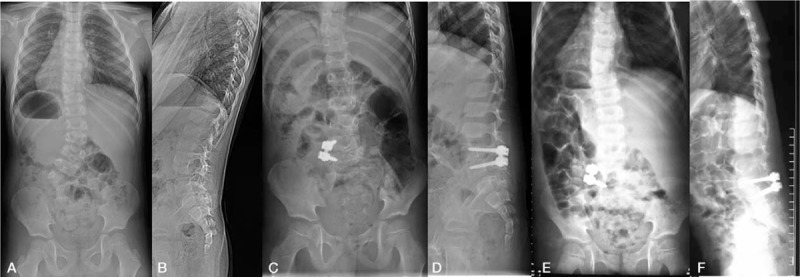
The patient was a 5-year-old boy with L5 hemivertebrae. One-stage posterior only approached hemivertebrae excision was performed. Reasonable radiological outcome was observed at follow up. A and B: Preoperative full spine radiographs showed the segmental curve was 43°, the compensatory lumbar curve is 22°. The coronal trunk balance was good. C and D: postoperative full spine radiographs showed the segmental curve was 8°, the compensatory lumbar curve is 15°. E and F: the full spine radiographs at final follow up showed the segmental curve was 9°, the compensatory lumbar curve is 13°.
